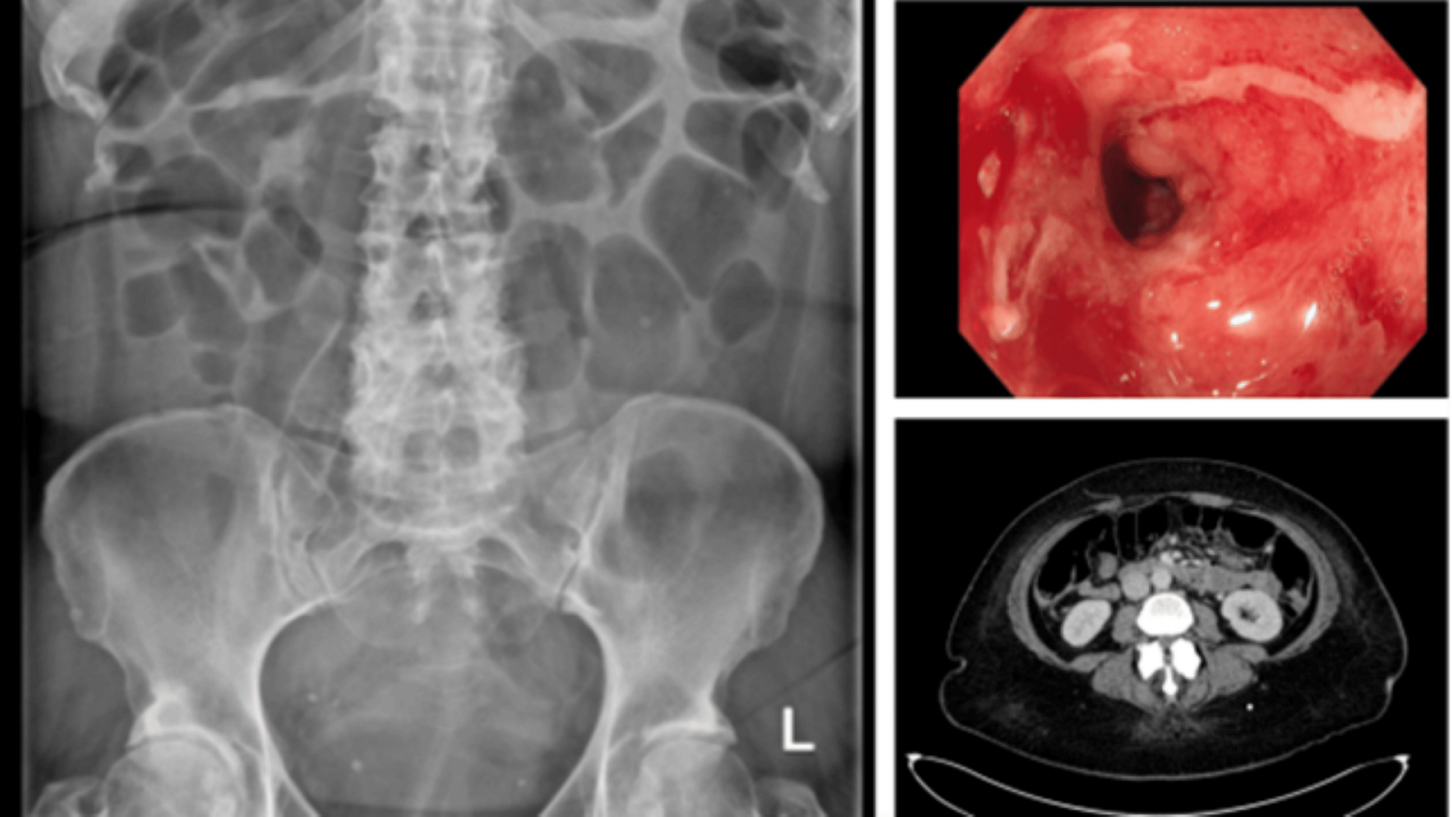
Abdominal X-ray and sigmoidoscopy Left: Plain abdominal X-ray demonstrating dilated bowel loops, specifically in the colon, suggestive of colitis.  Right-top:  Image from sigmoidoscopy confirming colitis. Right-bottom: CT image confirming colitis and the pattern of involvement.
